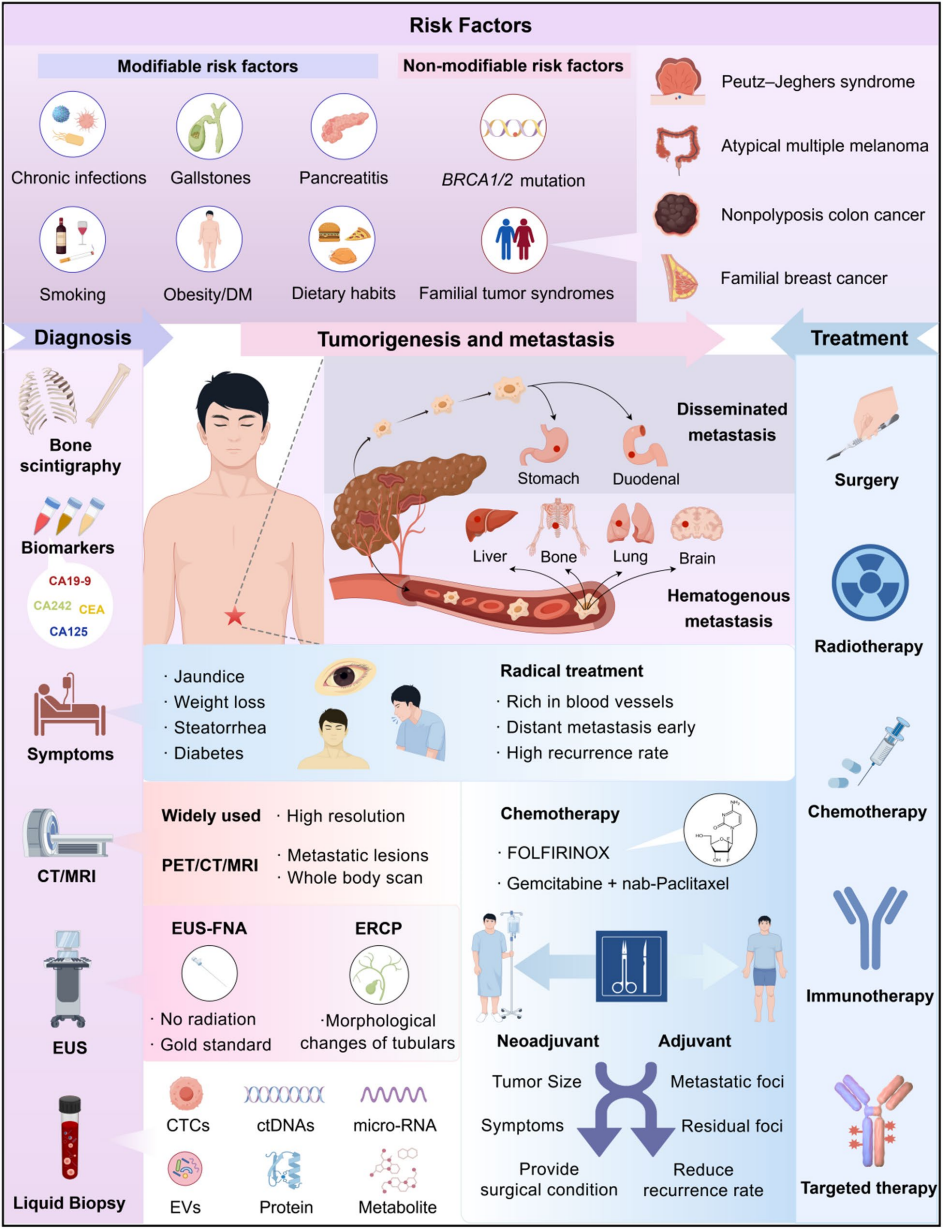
Comprehensive overview of PC diagnosis and treatment. In recent years, significant advancements have been made in diagnostic technologies for PC. In addition to traditional imaging techniques and pathological diagnosis methods, liquid biopsies and artificial intelligence methods are gradually being integrated into clinical practice. Treatment options for PC vary, with recent clinical studies highlighting the importance of individualized and precision treatments. Long-term follow-up management for PC patients is also essential, as it can influence therapeutic outcomes and enhance patients’ quality of life. Abbreviations: CT, computed tomography; MRI, magnetic resonance imaging; EUS, endoscopic ultrasonography; ERCP, endoscopic retrograde cholangiopancreatography; CTCs, circulating tumor cells; ctDNA, circulating tumor DNA; EVs, extracellular vesicles. The figure was created by Figdraw (www.figdraw.com)
